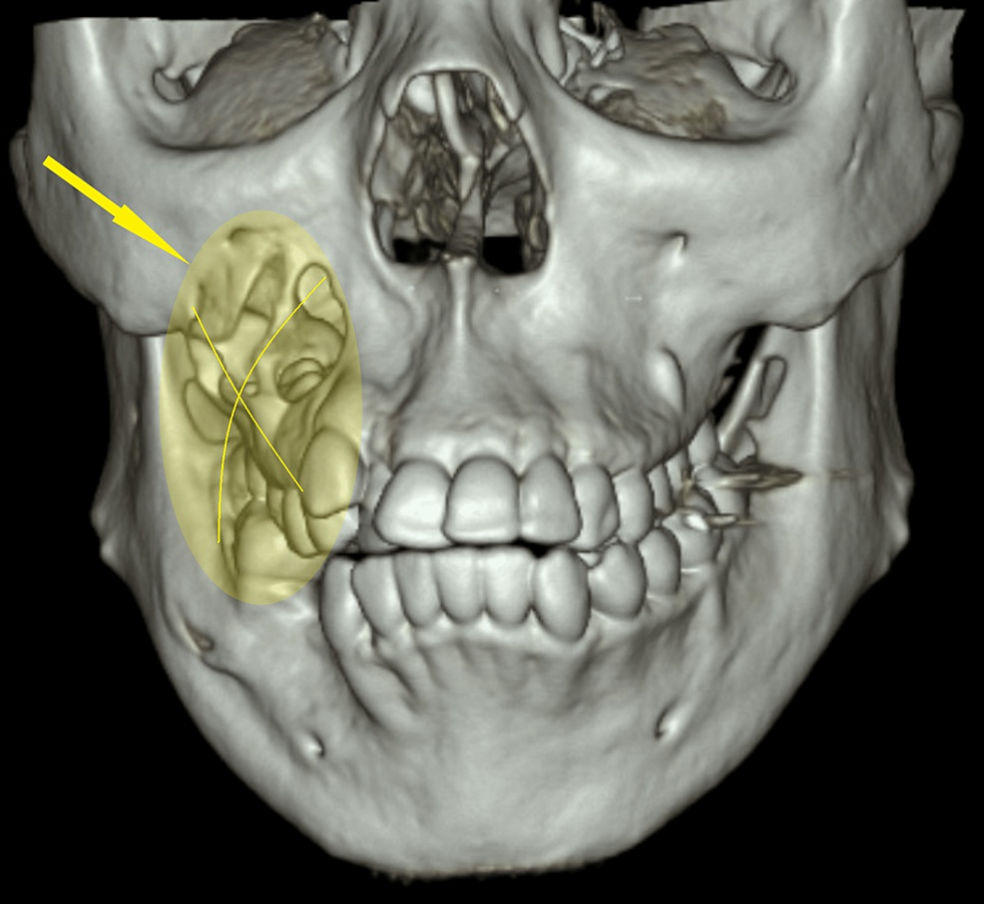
CT maxillofacial w/o contrast: 3D view 3.4 cm × 3.4 cm dentigerous cyst of the right maxillary sinus with expansion thinning of the surrounding maxillary sinus walls.
